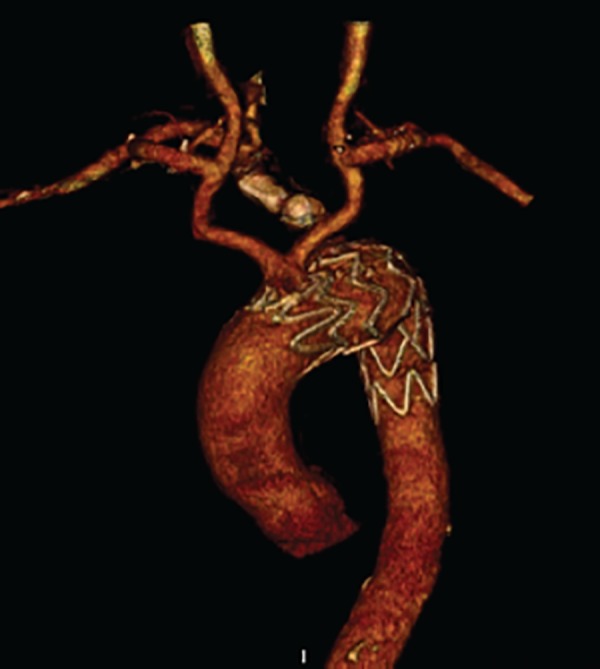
Six Month after operation CT-A 3D reconstruction
